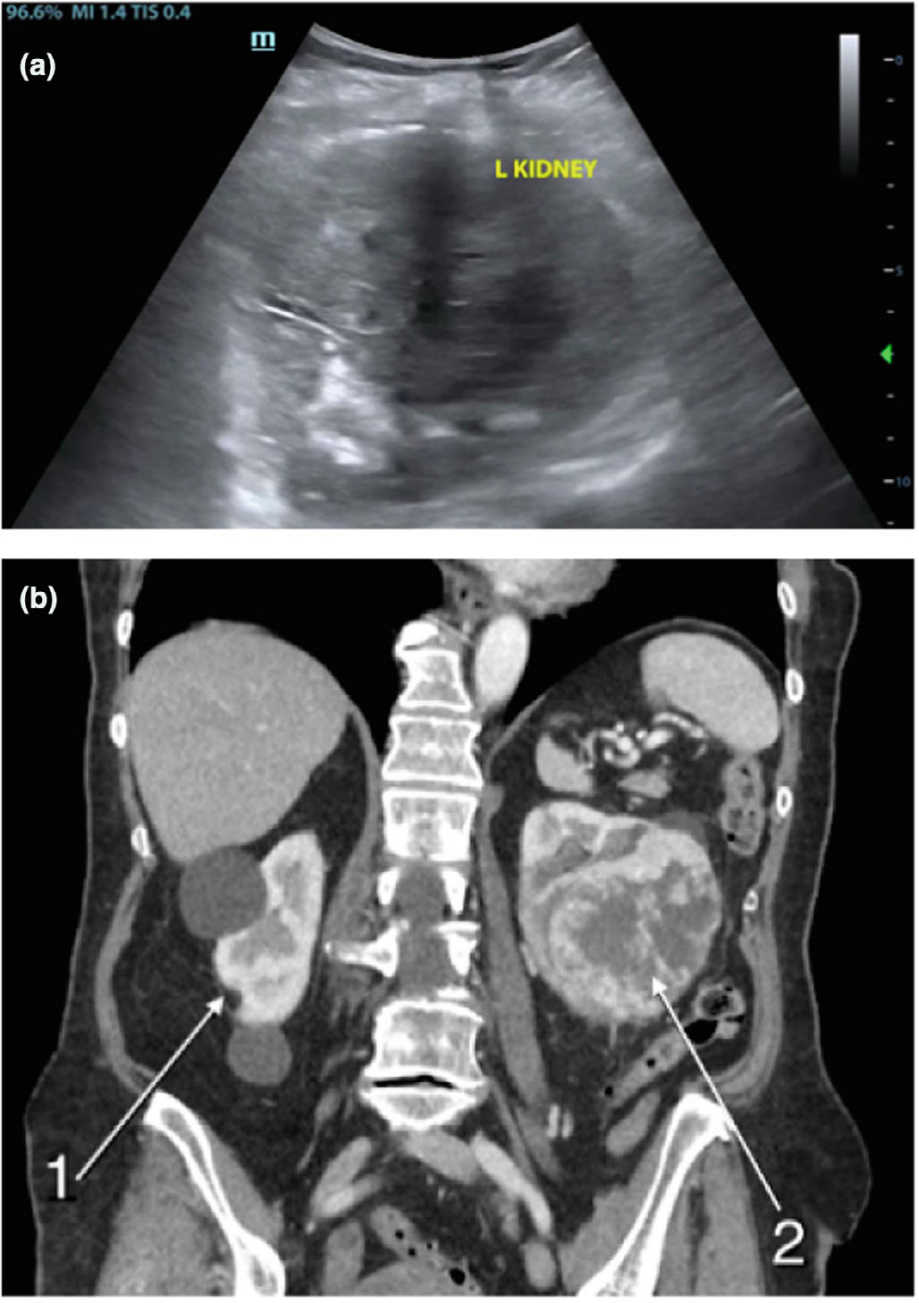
Renal cell carcinoma in the left kidney of an 83 year old woman. (a) Point‐of‐care ultrasound showing an abnormal hypoechoic region in the left renal cortex suspicious for malignancy. (b) Computed tomography scan of the abdomen and pelvis showing a normal‐appearing right kidney (1) with two renal cysts and a left renal mass (2) consistent with renal cell carcinoma.
